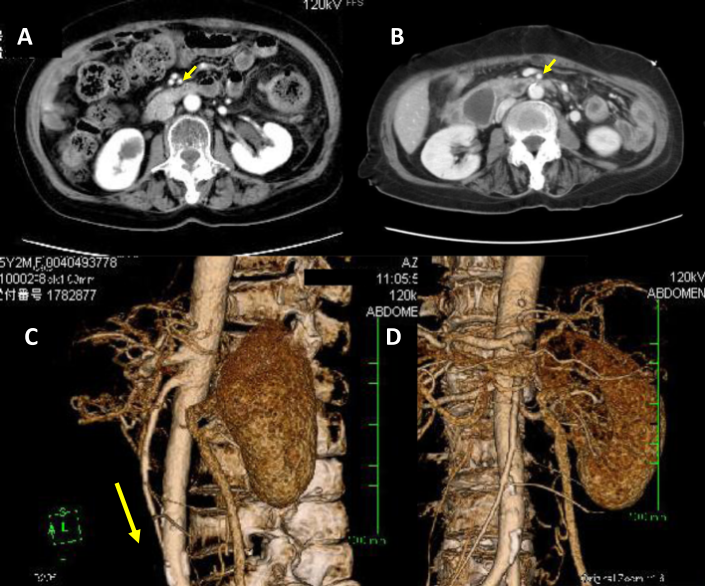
CT shows a narrow angle and reduced distance between the aorta and SMA. (A) The distance between the aorta and SMA was 12 mm on preoperative CT. (B) The distance between the aorta and SMA on postoperative CTwas 2mm. (C, D) The angle between the aorta and SMA on postoperative CT was 36°. Localization of the pouch deep in the pelvis causes relative stretching of the SMA and flattens it against the aorta in the retroperitoneum.
